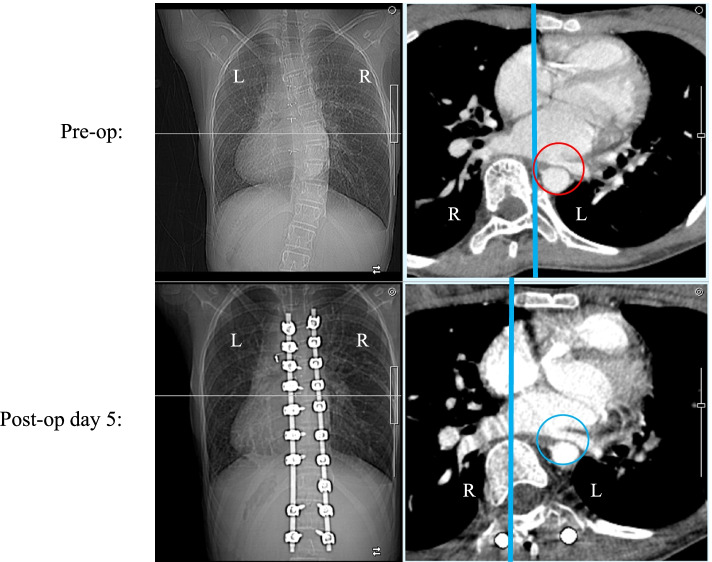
Changes in pre- and postoperative contrast CT. A midline and posterior shift of the T8 vertebral body relative to the sternum (blue circle) as compared with preoperative images (red circle)
